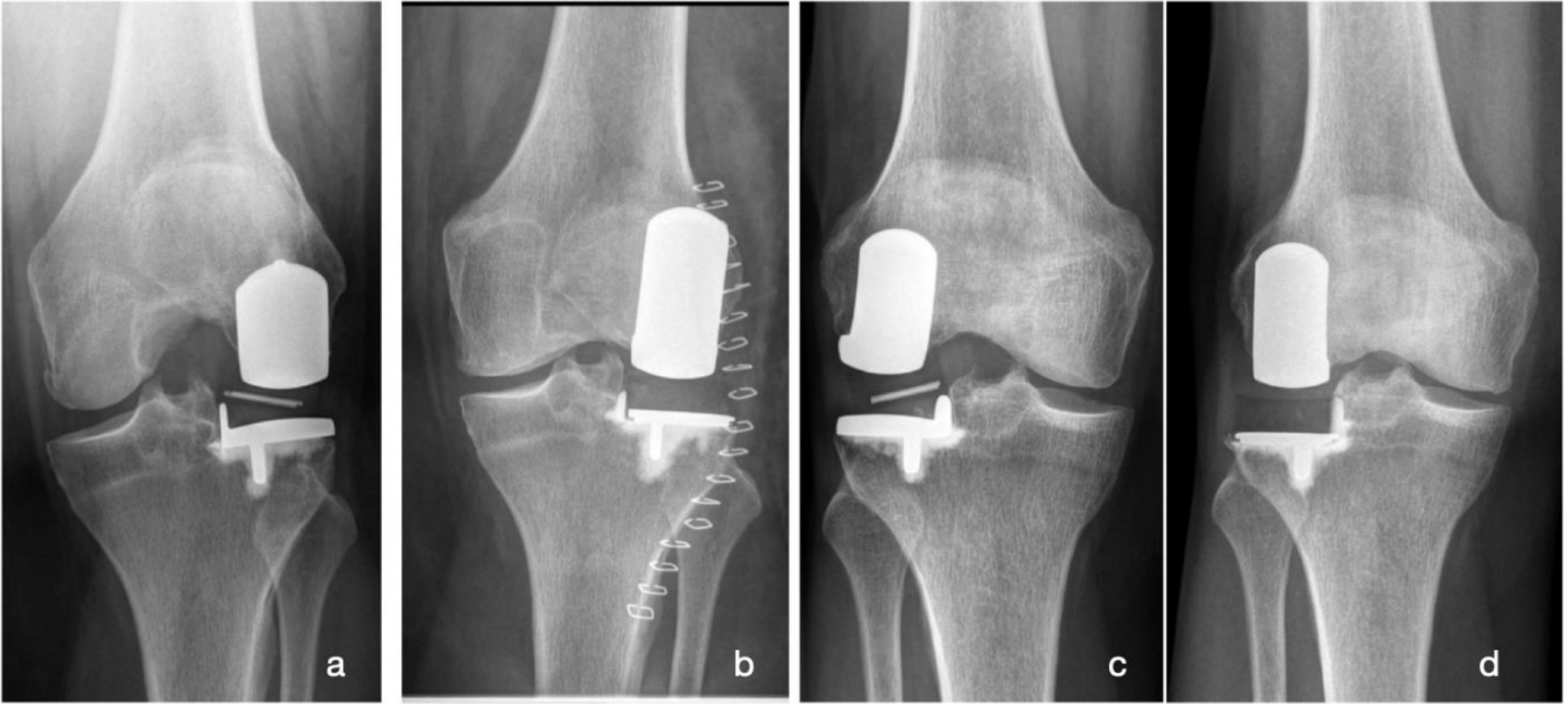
Radiographs of two female patients who have experienced medial bearing dislocation after 46 (a) and 60 (c) months, respectively. Both patients received an exchange of the tibial component to a fixed-bearing design (b: Oxford Fixed Lateral; d: Vanguard M Partial). a and c: AP x-rays with a dislocated bearing into the notch. b and d: Postoperative AP x-rays after revision to a fixed-bearing design. AP: antero-posterior
